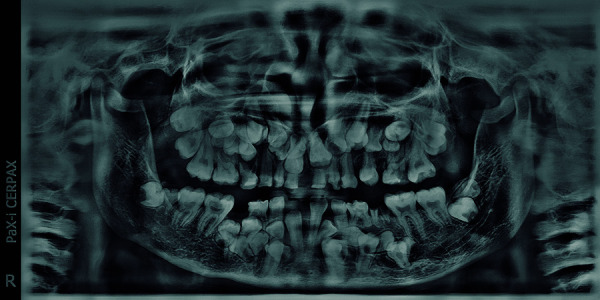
Panoramic radiograph showing multiple supernumerary teeth and parallel ascending branches.
